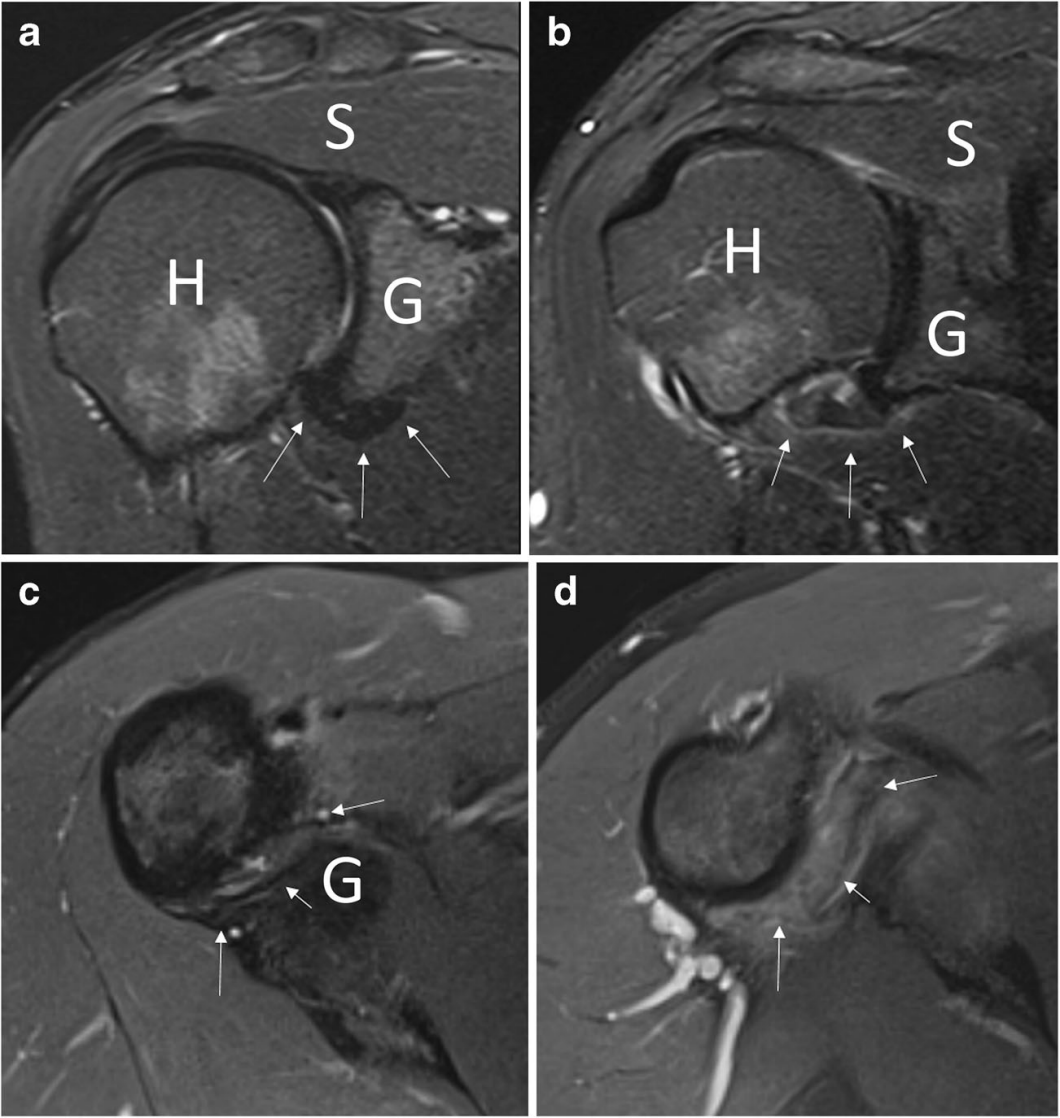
Coronal oblique T2-weighted fat-saturated (a, b) and axial proton density fat-saturated (c, d) images. In a healthy subject (a, c), the capsular recess has normal signal intensity (*arrows*), while in a patient with adhesive capsulitis (b, d), clear signal hyperintensity can be seen (*arrows*). *H* humerus, *G* glenoid, *S* supraspinatus tendon
